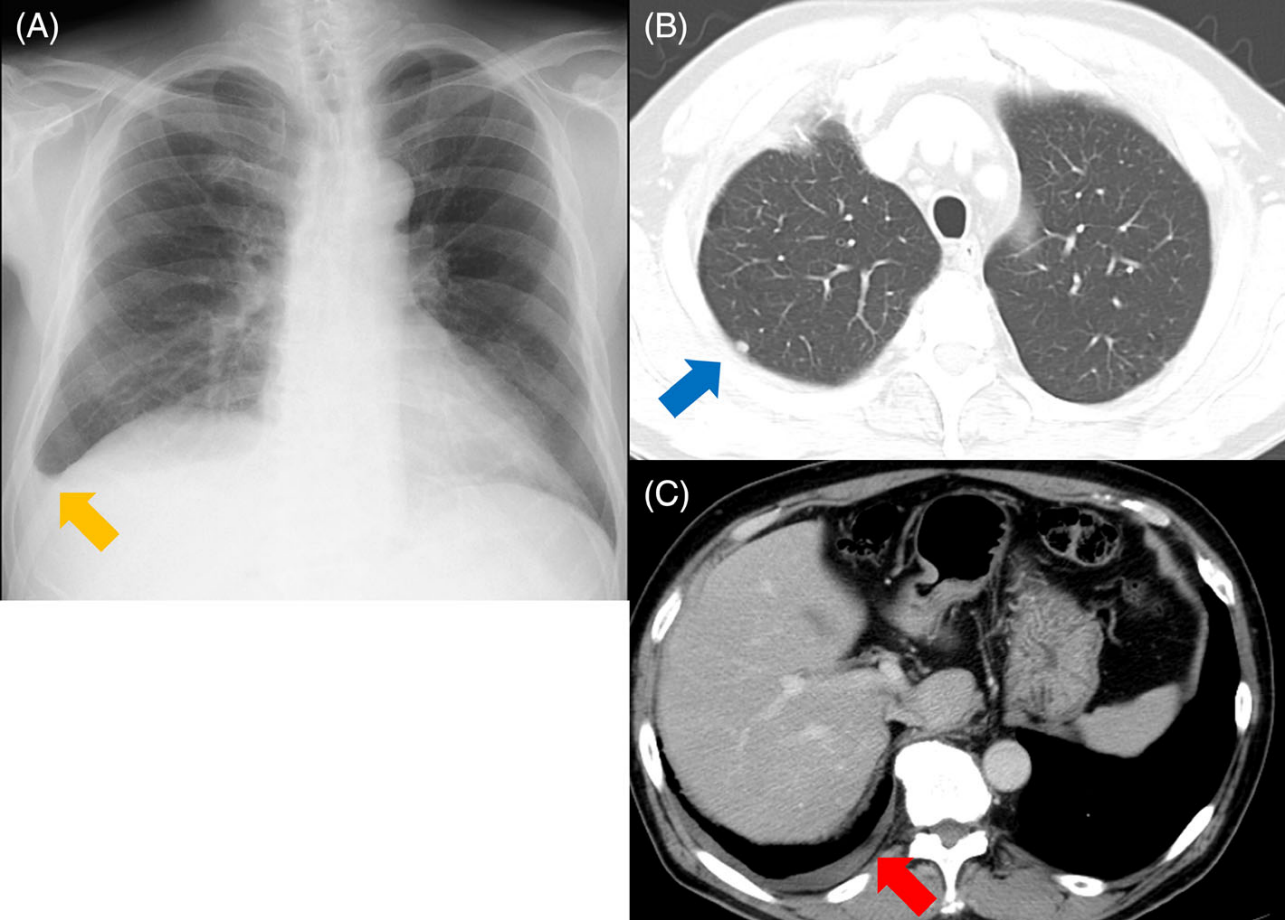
Chest x‐ray showed right pleural effusion (yellow arrow) (A). Chest computed tomography showed a nodular shadow bordering the pleura (blue arrow) (B) and right pleural effusion (red arrow) (C).
